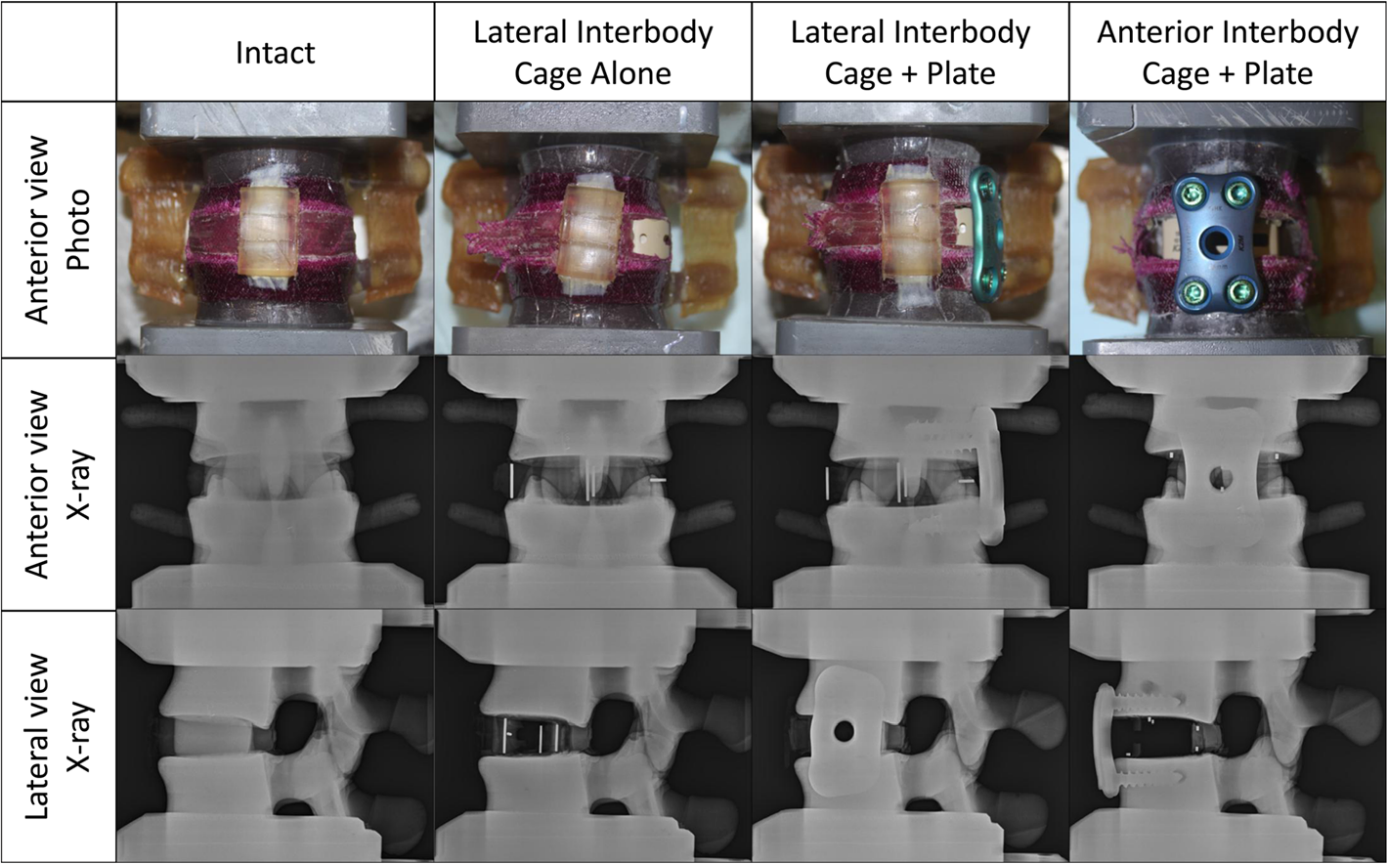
Anterior view photo, anterior view x-ray and lateral view x-ray imaging of different sample conditions.
